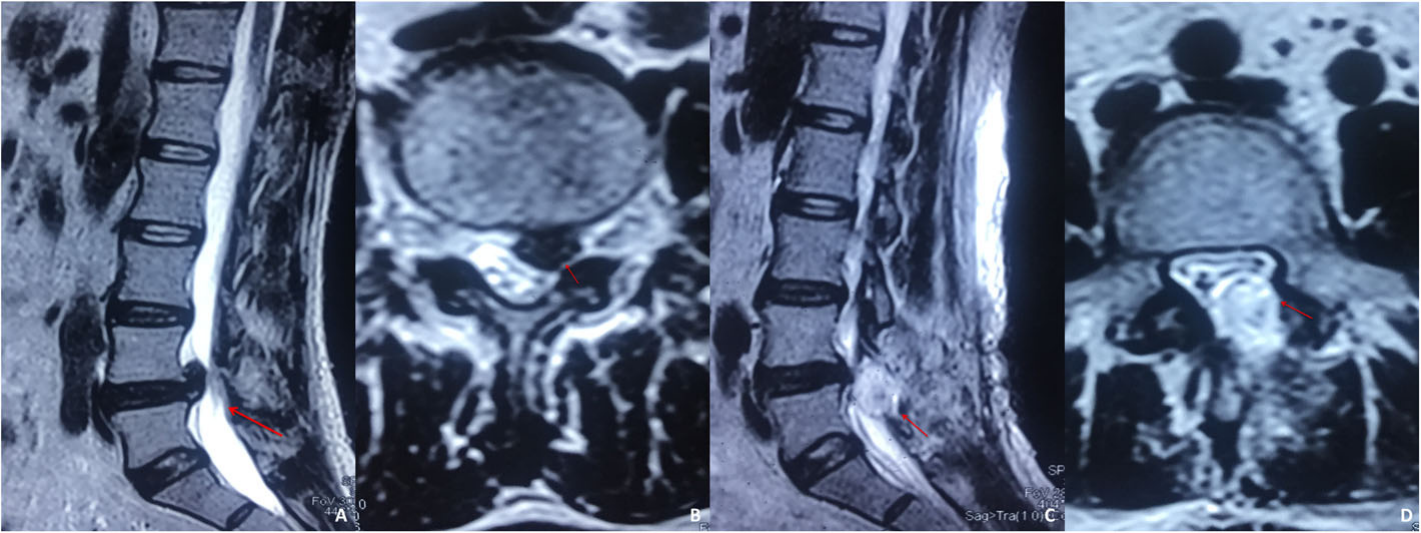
Only one patient had symptomatic epidural haematoma. A 37-year-old male with a herniated disc at the L4/5 segment required discectomy treatment. (a) Sagittal MRI showed the L4/5 segment with a substantially herniated disc. (b) Transverse MRI showed herniated nucleus pulposus tissue, resulting in compression of the left nerve root. (c d) The symptoms of left lower limb pain recurred after discectomy. The sagittal and transverse MRI sections showed haematoma formation, and the dural sac and left nerve root were obviously compressed
